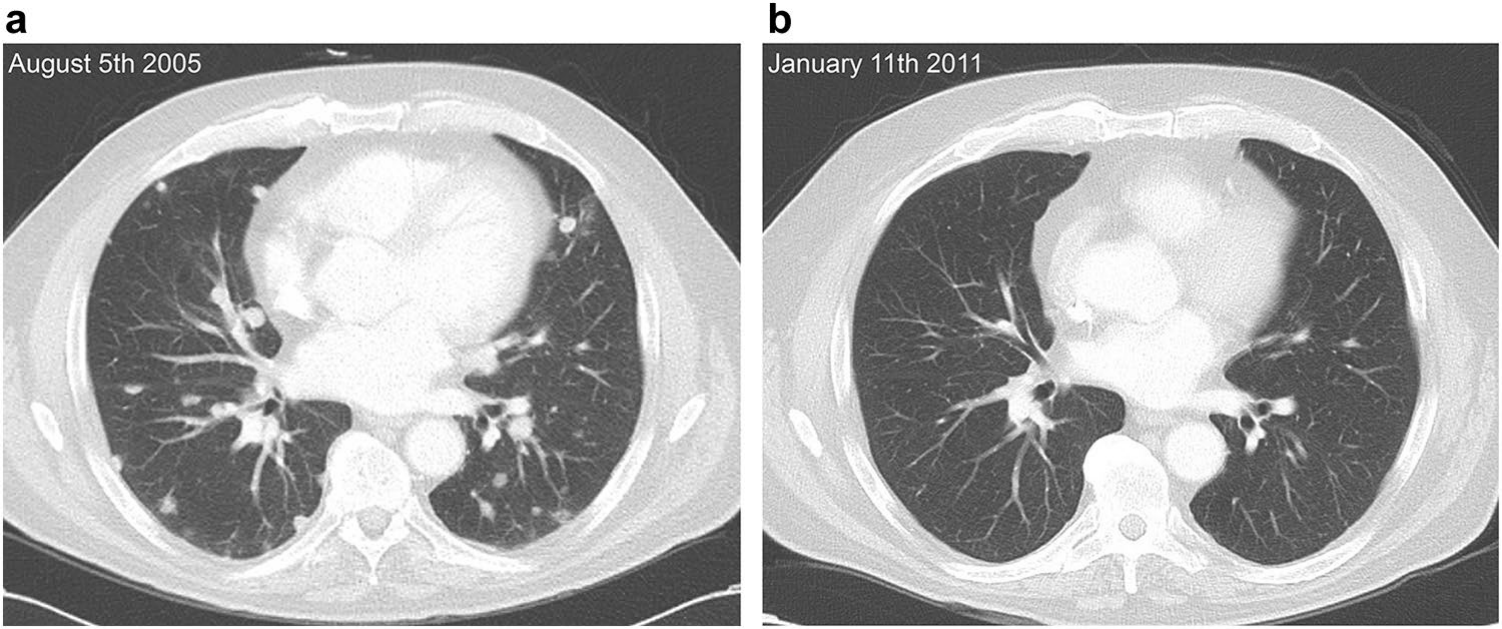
Pre-treatment lung window CT scan of the patient that had a complete response shows multiple solid nodules in both lungs (a). In the most recent lung window CT scan (approximately, 5 and a half years later), no solid lung nodules are identified (b)
